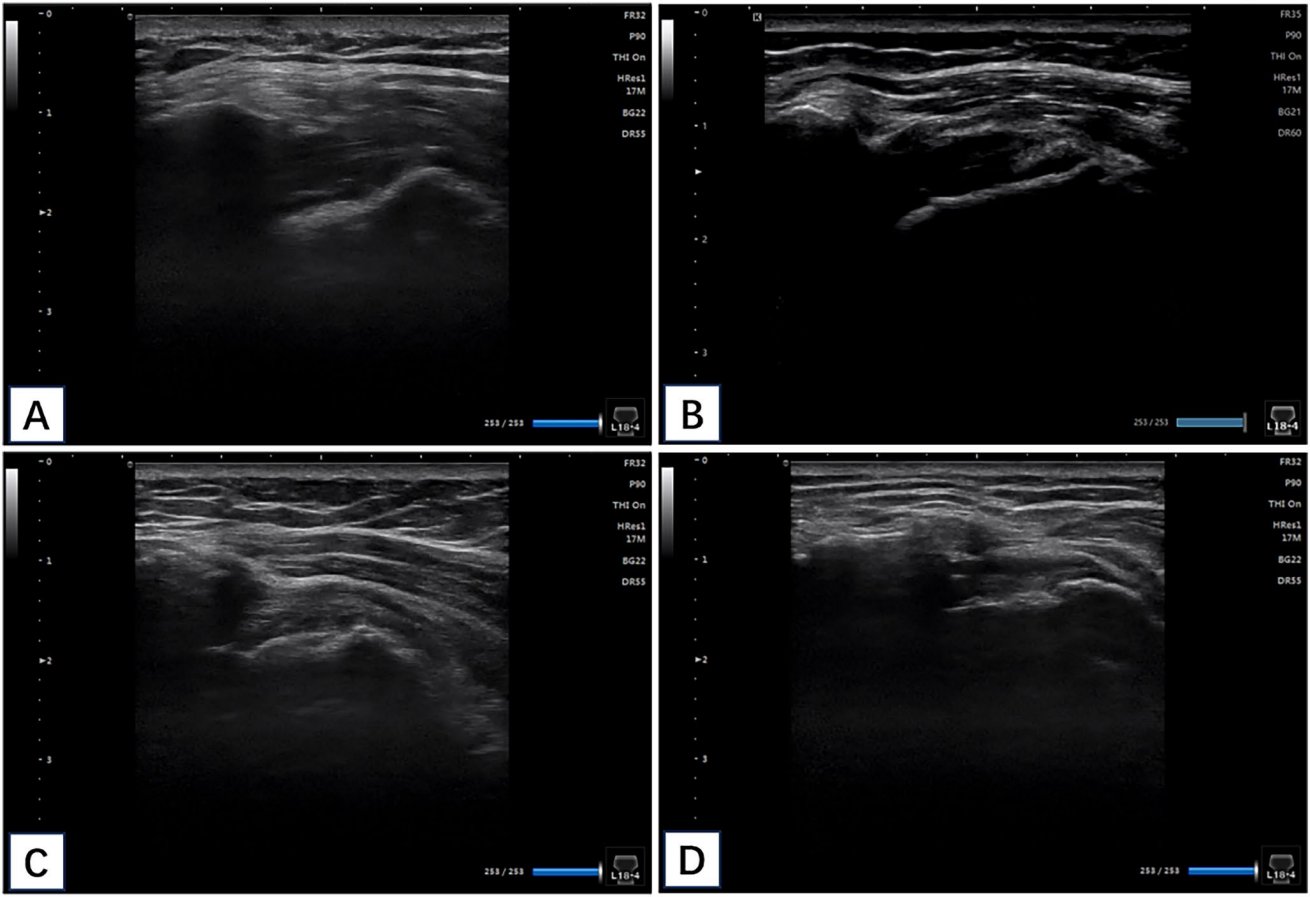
Postoperative ultrasound imaging. (A) Retained group, Grade I: Intact tendon repair with sufficient thickness. Clinical outcome: ASES = 90, VAS = 1. (B) Retained group, Grade IV: Focal full‐thickness tear indicating structural failure. Clinical outcome: ASES = 75, VAS = 3. (C) Tenotomy group, Grade I: Intact tendon repair with sufficient thickness and homogeneous low echogenicity. Clinical outcome: ASES = 95, VAS = 1. (D) Tenotomy group, Grade IV: Small, focal full‐thickness tear. Clinical outcome: ASES = 85, VAS = 2. ASES, American Shoulder and Elbow Surgeons; VAS, visual analog scale.
